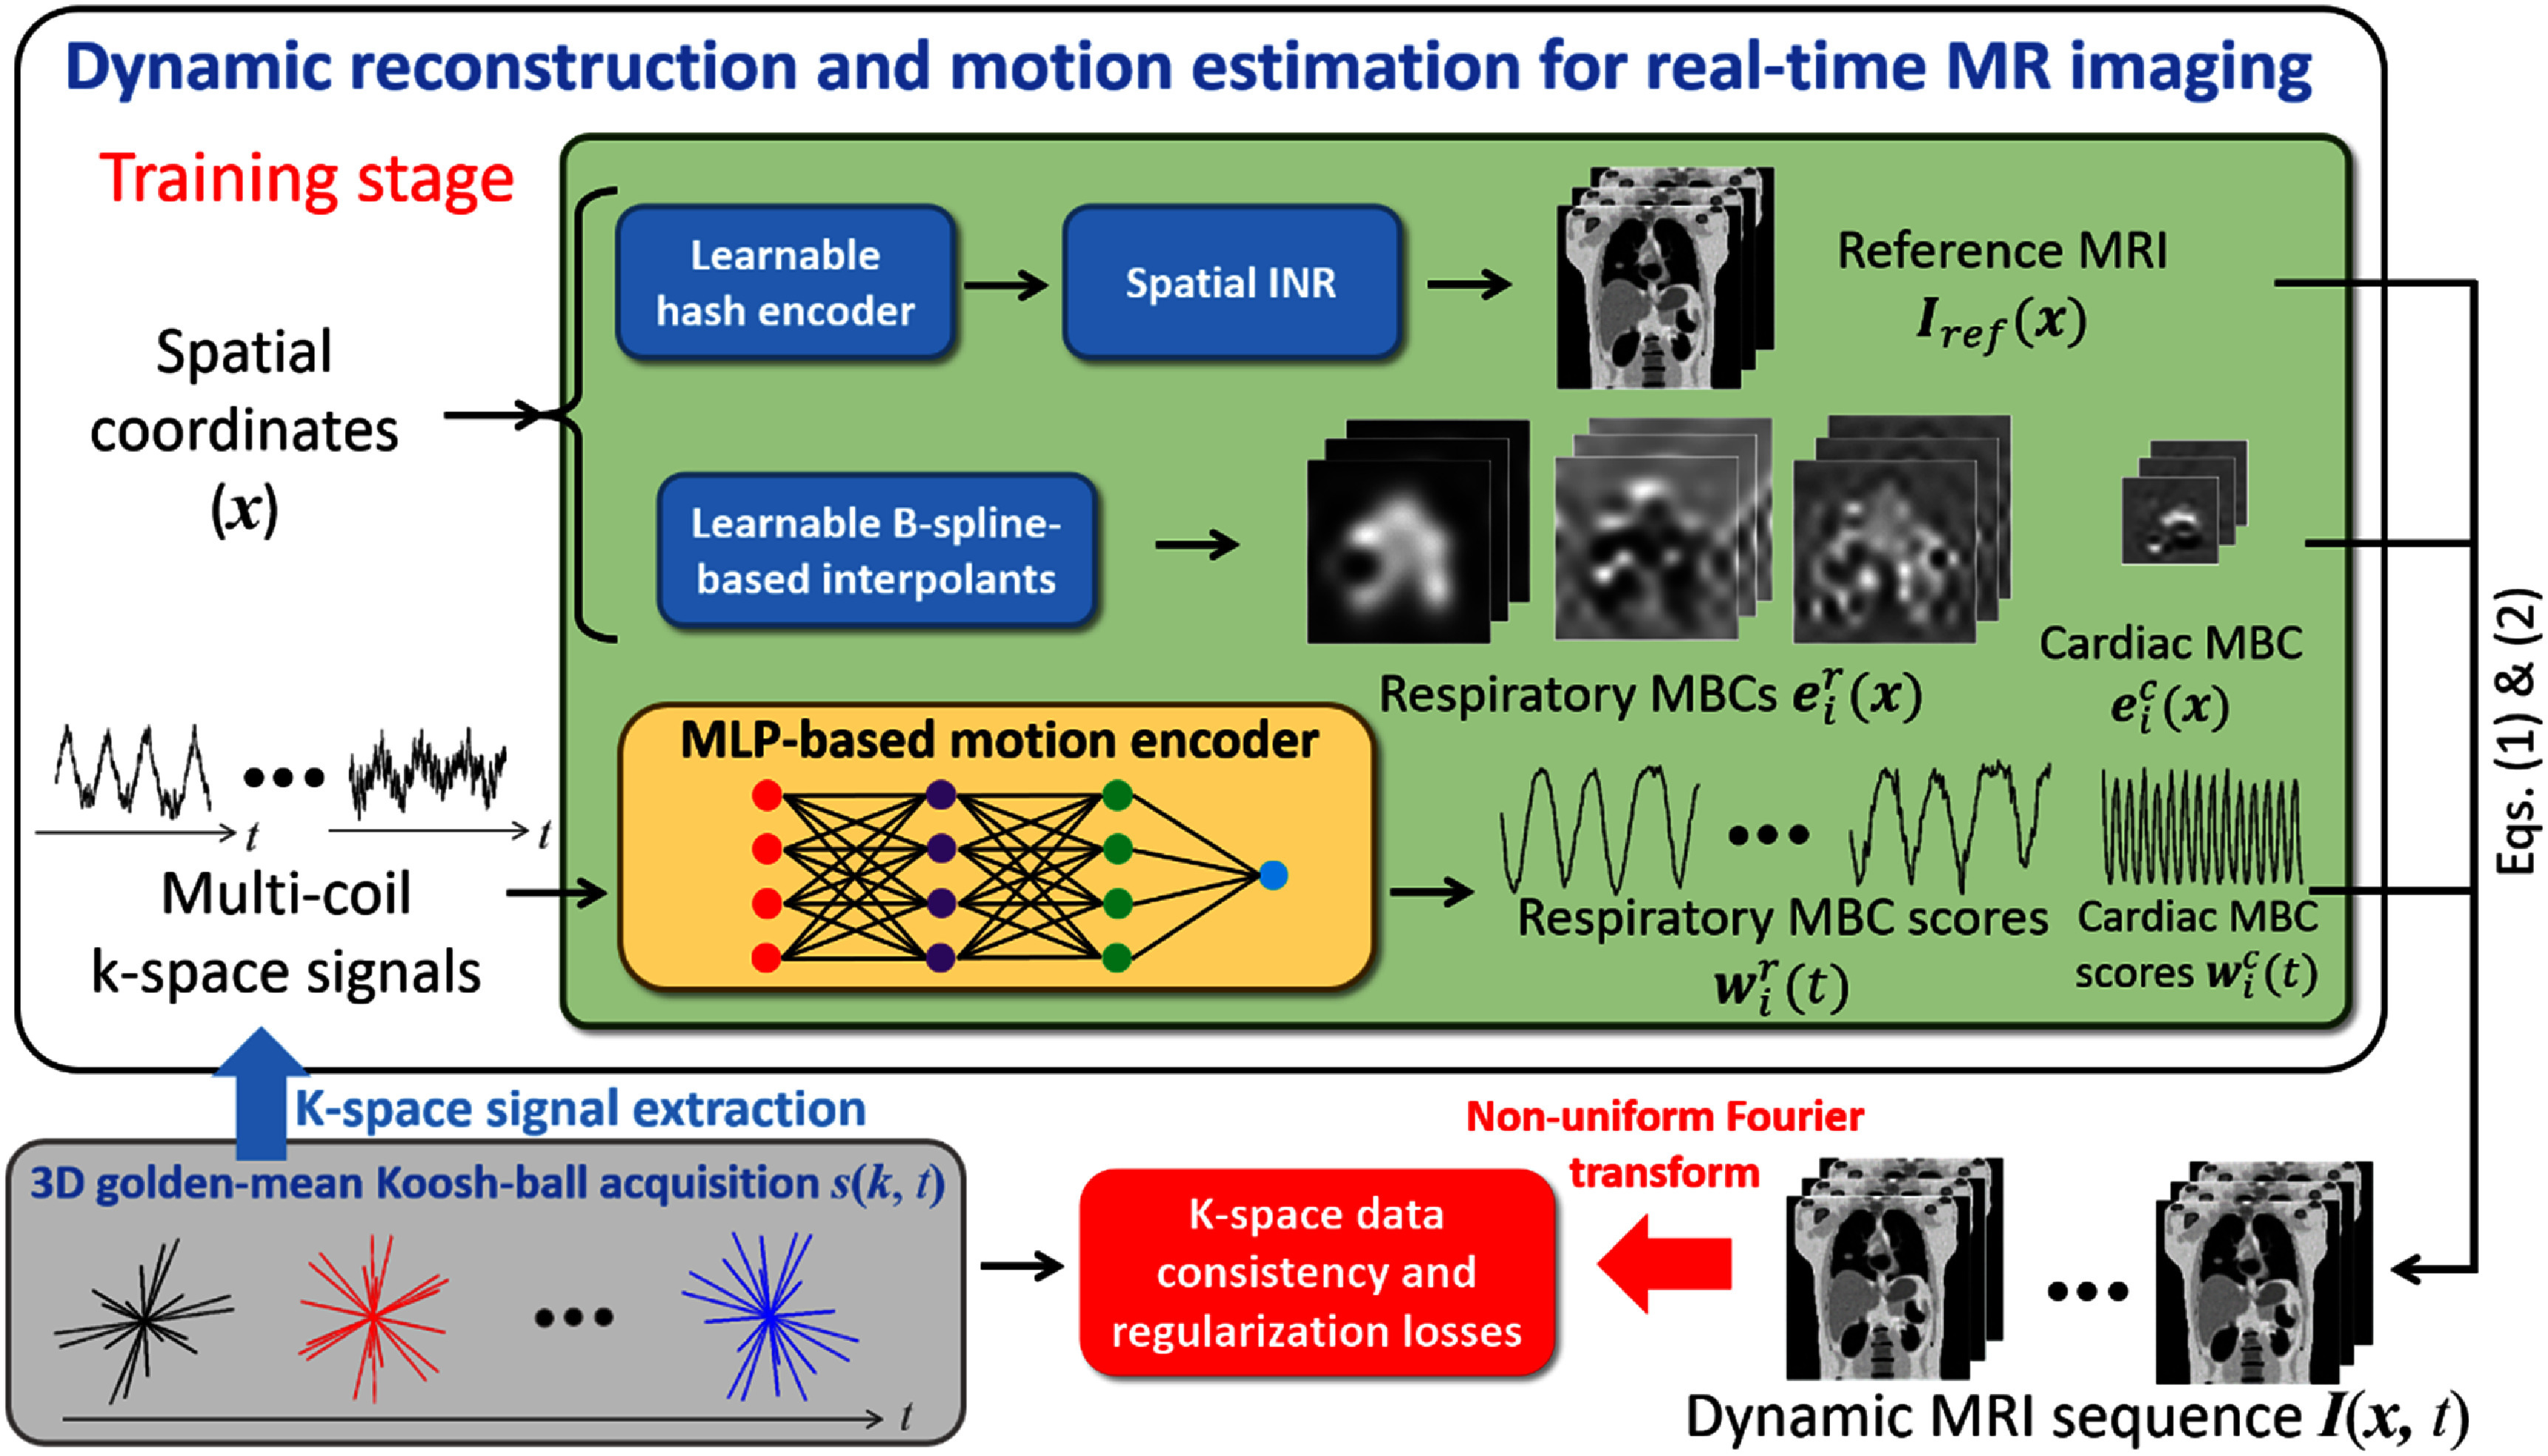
Overview of the DREME-MR framework and the dual-task learning strategy. DREME-MR simultaneously reconstructs a dynamic sequence of volumetric MR images (learning task 1) and trains a multilayer perceptron (MLP)-based motion encoder for real-time imaging and motion tracking (learning task 2), using a pre-treatment MR scan. DREME-MR adapts a joint reconstruction and deformable registration approach for dynamic volumetric MRI reconstruction. Specifically, it reconstructs a reference 3D anatomy ${\boldsymbol{I}_{\text{ref}}}\left( {\boldsymbol{x}} \right)$ and solves a cardiorespiratory-resolved dynamic motion field ${\boldsymbol{d}}\left( {\boldsymbol{x},{\text{ }}t} \right)$ with respect to ${\boldsymbol{I}_{\text{ref}}}\left( {\boldsymbol{x}} \right)$ to generate dynamic MRIs ${\boldsymbol{I}}\left( {\boldsymbol{x},{\text{ }}t} \right)$. The reference anatomy ${\boldsymbol{I}_{\text{ref}}}\left( {\boldsymbol{x}} \right)$ is solved by the spatial implicit neural representation (INR). The dynamic motion fields ${\boldsymbol{d}}\left( {\boldsymbol{x},{\text{ }}t} \right)$ are decomposed into spatial ${\boldsymbol{e}_i}\left( {\boldsymbol{x}} \right)$ and temporal ${\boldsymbol{w}_i}\left( t \right)$ components which are separately estimated by learnable B-spline interpolants and an MLP-based motion encoder, respectively. The motion encoder estimates the time-varying motion coefficients directly from the multi-coil *k*-space signals extracted from MR signals ${\boldsymbol{s}}\left( {\boldsymbol{k},{\text{ }}t} \right)$ acquired by a 3D golden-mean Koosh-ball trajectory. Therefore, it can be directly applied to the subsequent treatment session for real-time motion estimation, using online time series of MR signals. The dual-task learning is driven by a *k*-space data consistency loss and regularization losses. MBC: motion basis component.
